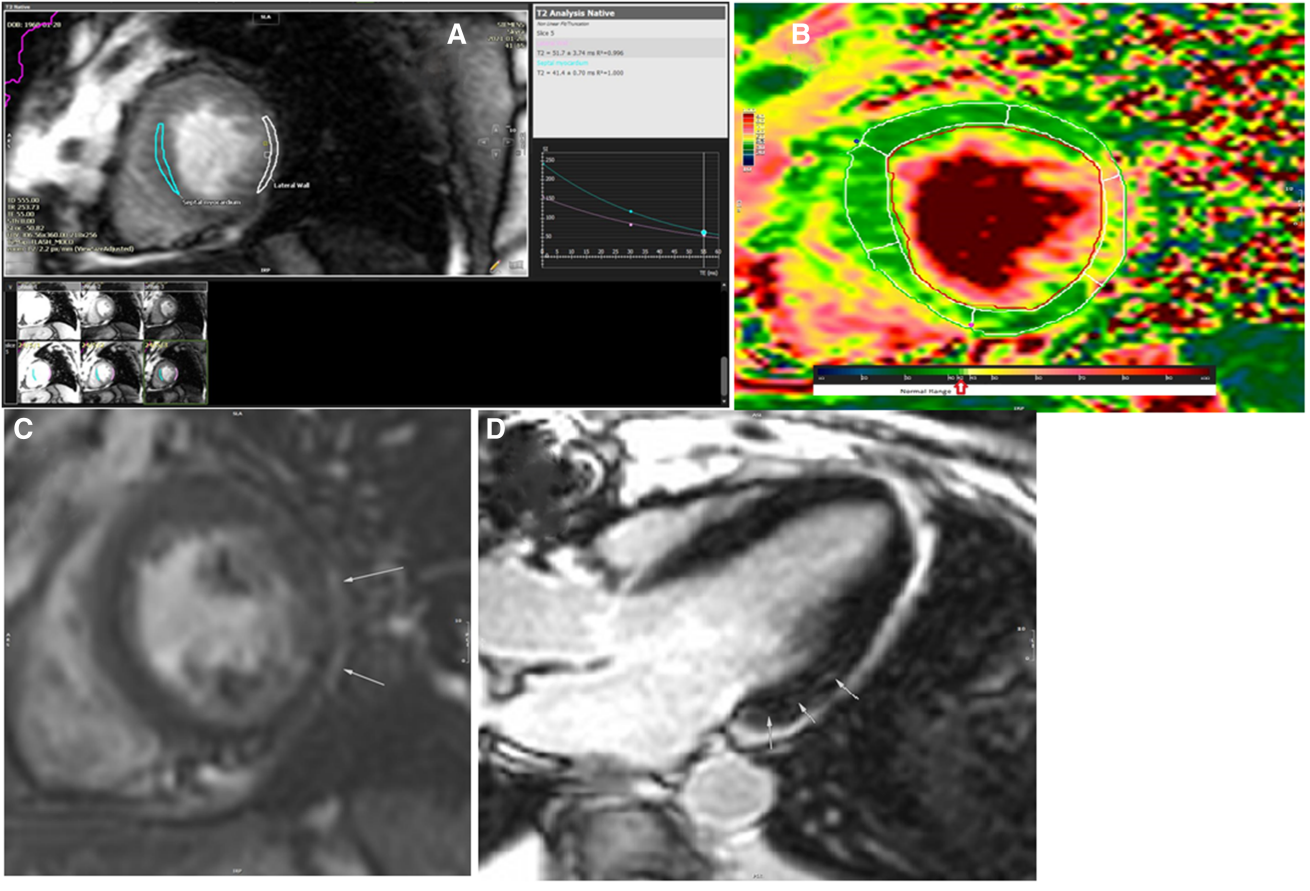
The results of cardiac magnetic resonance imaging after the radiation (A–D). (A) T2 analysis of mid-septal and mid-lateral segments of the left ventricle revealed prolongation of T2 relaxation time in the mid to apical segments of the lateral wall (52 ms). (B) T2 map image of the left ventricle in short axis view showed abnormal T2 value in the mid-lateral segment of the left ventricle. (C) Delayed postcontrast image of the left ventricle in short axis view showed a subepicardial rim of late gadolinium enhancement on the lateral wall of the left ventricle. (D) Delayed postcontrast image of the left ventricle in long axis three-chamber axis view revealed small focal subepicardial to mid-wall patches of late gadolinium enhancement in the basal inferolateral segment.
